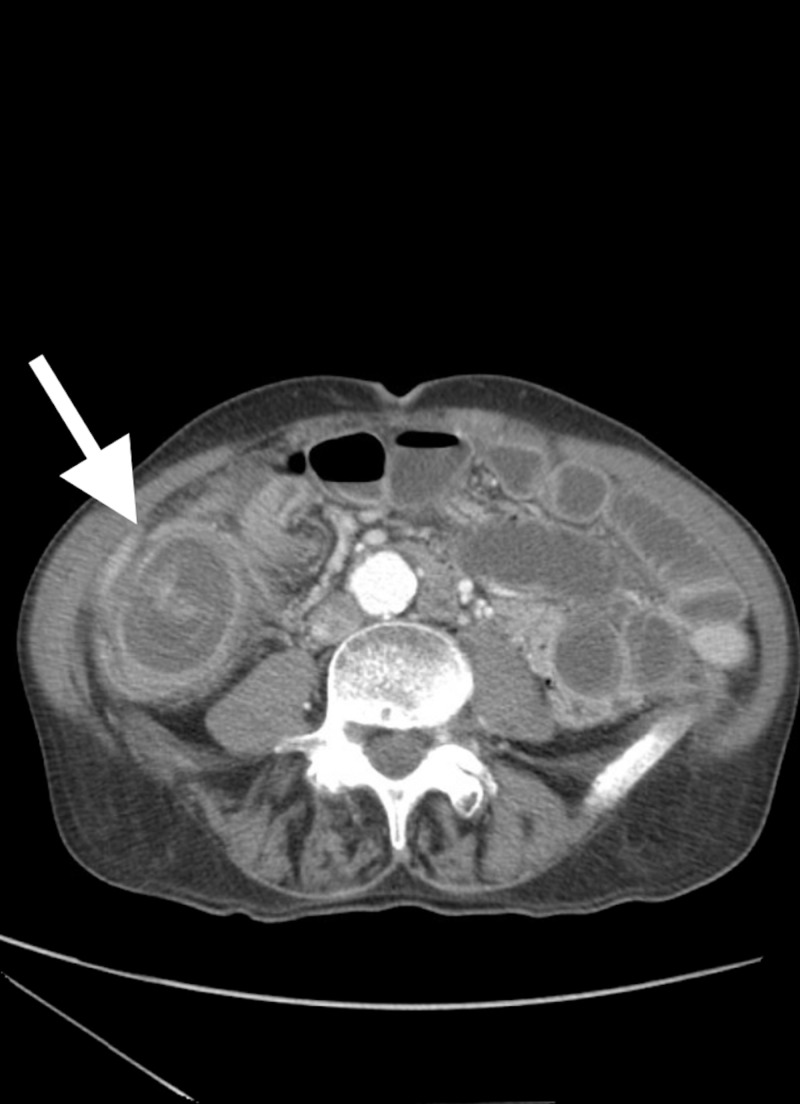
Axial computed tomography (CT) scan showing intussusception
